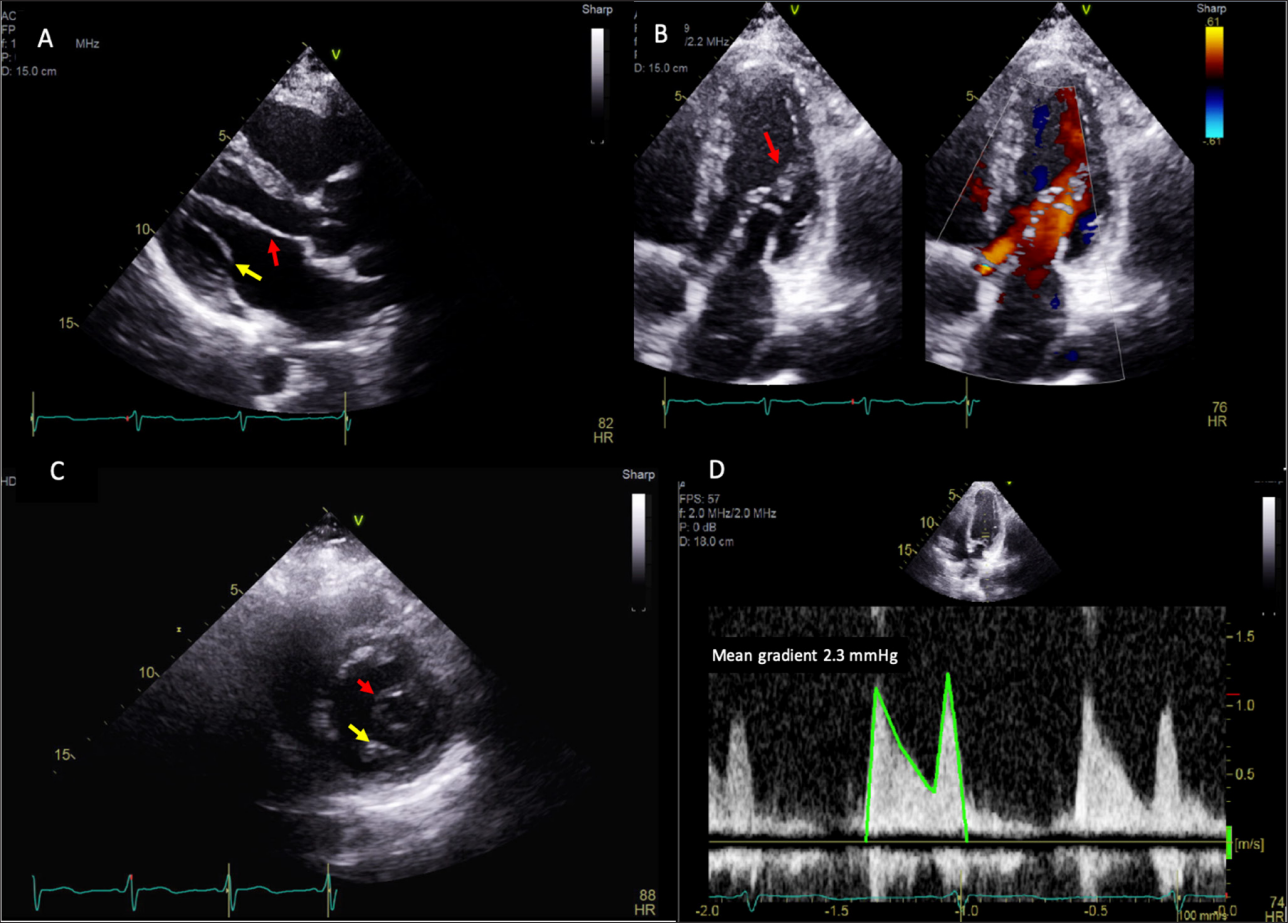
(A) Parasternal long view on transthoracic echo showing thickened anterior (red arrow) and posterior mitral valve (yellow arrow) leaflets. (B) Apical long-axis view showing pear-shaped eccentric opening of the mitral valve with short chordae tendinea attached to the anterolateral papillary muscle (red arrow) and transmitral flow during diastole. (C) Parasternal short-axis view showing an eccentric mitral valve orifice (red arrow) predominately oriented towards the anterolateral papillary muscle and a remnant of the posteromedial papillary muscle (yellow arrow). (D) Spectral Doppler showing mean gradient of 2.3 mm Hg at a heart rate of 74 beats/min across the mitral valve in diastole.
